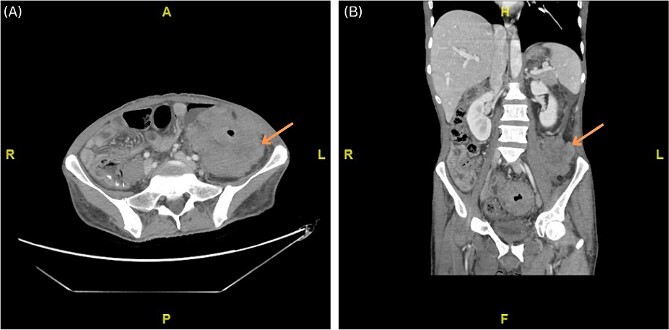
(A, B) Axial and coronal computed tomography (CT) scan showing marked non-uniform wall thickening and nodularity with heterogeneous enhancement invading the lateral part of the left psoas muscle and the left transverse abdominis muscle.
